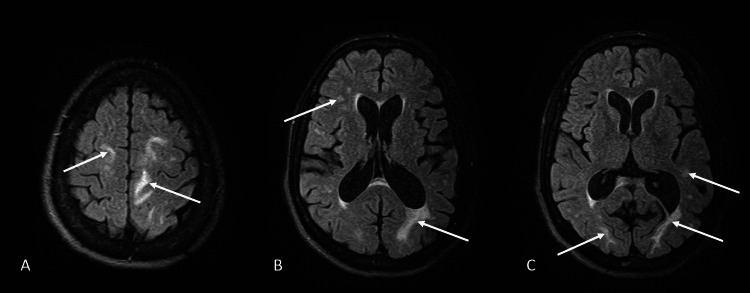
Magnetic resonance imaging of the brain in a patient with pregabalin-induced PRES (A) Axial fluid-attenuated inversion recovery (FLAIR) image showing hyperintensities in bilateral frontal lobes (arrows). (B and C) Axial FLAIR images showing hyperintensities in the right frontal lobe, left parietal lobe, and bilateral occipital lobes (arrows). PRES = posterior reversible encephalopathy syndrome
